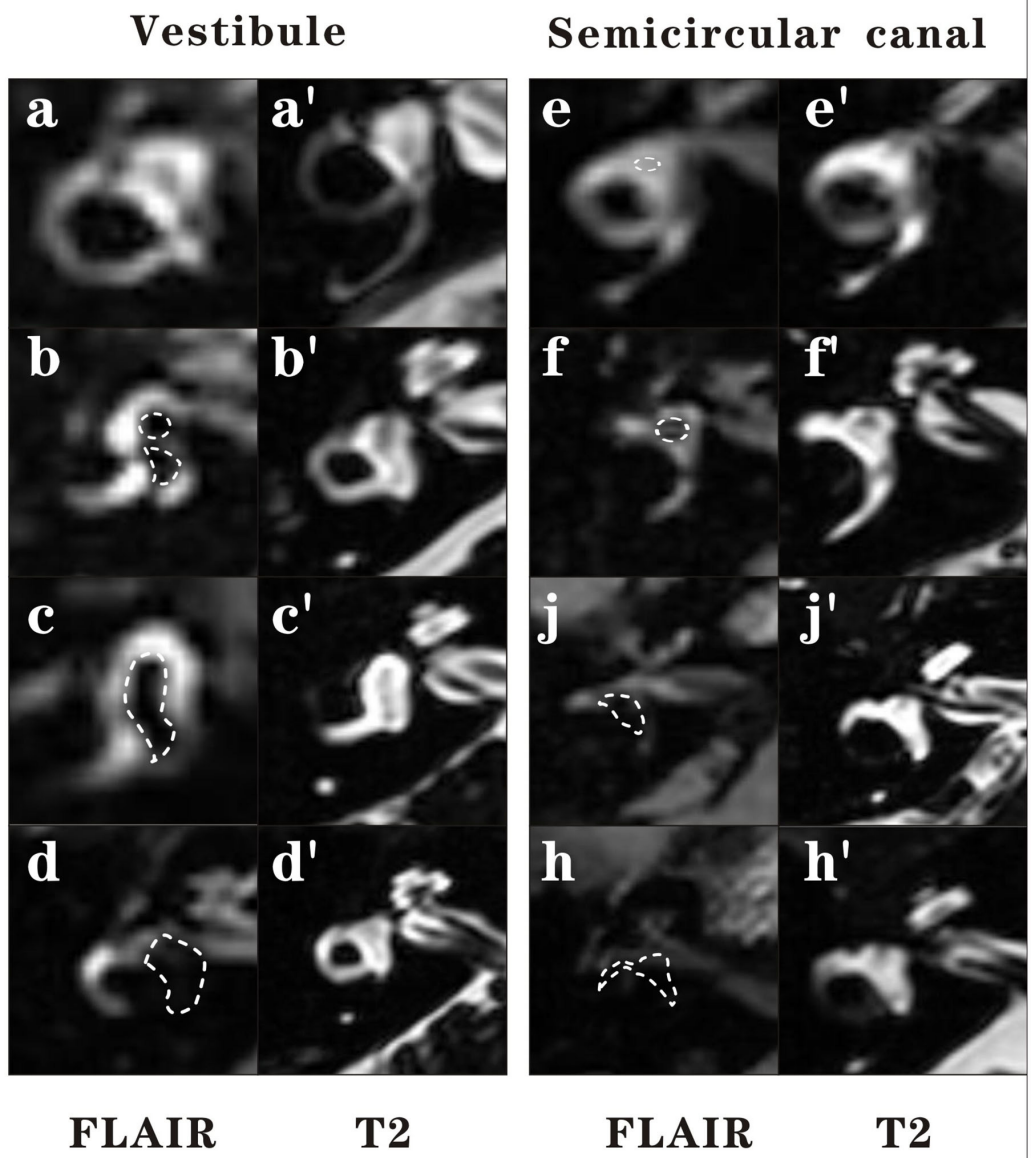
Four grades of the vestibular EH and lateral semicircular canal EH. In this figure, 3D-FLAIR pictures (a–h) are shown adjacent to 3D-T2-SPAIR pictures (a′-h′) for each grade. For vestibule, grade 0 = No hydrops (a). The saccule and utricle are visibly separately and take less than half of the surface of the vestibule. Grade 1 = Mild hydrops (b). When the saccule become equal or larger than the utricle, but is not yet confluent with the utricle. Grade 2 = Moderate hydrops (c). There is a confluence of the saccule and utricle with still a peripheral rim enhancement of the perilymphatic space. Grade 3 = Severe hydrops (d). The perilymphatic enhancement is invisible. For lateral semicircular canal, grade 0 = No hydrops (e). A small visible herniation which is 1/3 less than the semicircular canal with perilymph surrounding can be observed. Grade 1 = Mild hydrops (f). A dark area occupying over 1/3 of the ampulla is observed. Grade 2 = Moderate hydrops (g). The crura become dark and some of the canals become invisible with hydrops, which often accompany the stenosis of canals. Grade 3 = Severe hydrops (h). All semicircular canals were invisible.
